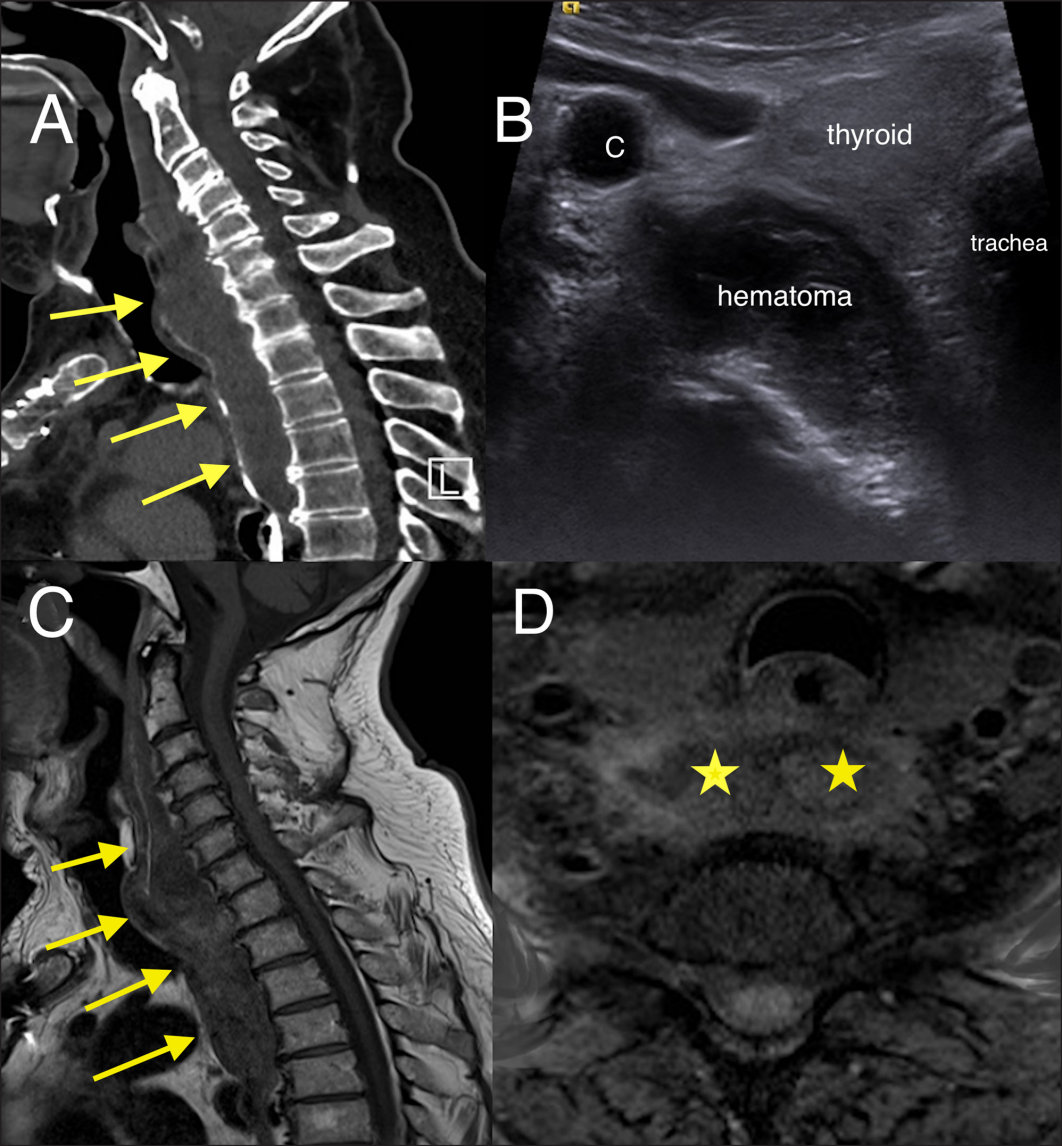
(A) Sagittal CT image with oral gastrografin administration showing a pre-vertebral hematoma displacing the pharynx (arrows). (B) Axial US image showing the slightly echoic collection pushing forward trachea and thyroid. (C) Sagittal T1-weighted image and (D) Axial T1-weighted image with fat saturation showing the retropharyngeal hematoma on day 7 with methemoglobin hyperintensity (arrows and asterisks).
